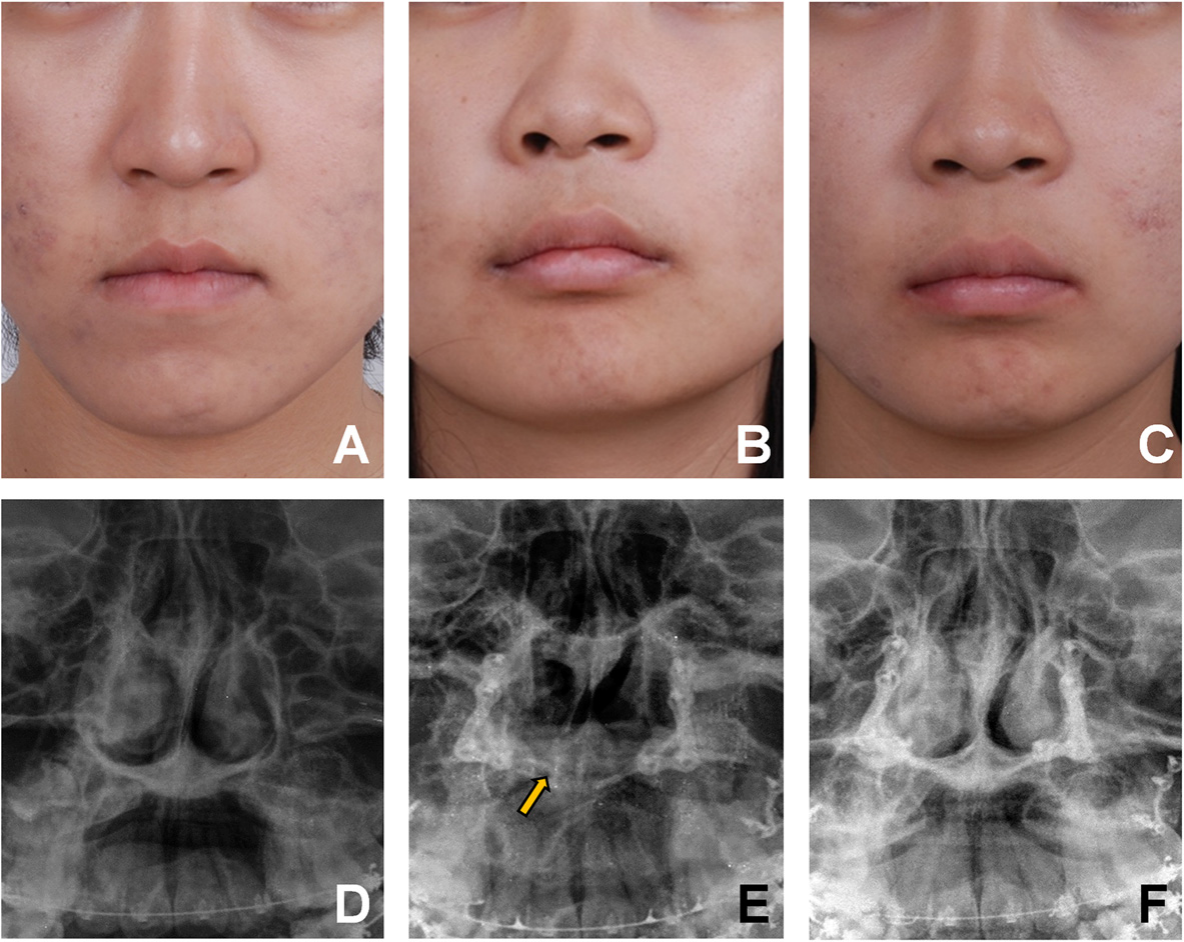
Presurgical condition of case 2 patient before Le Fort I osteotomy (a, d), immediate after the Le Fort I osteotomy (b). Deviated nasal septum (*arrow*) was visible in radiographic examination (e). After correction of nasal septum deviation (c, f). The patient showed corrected septal deviation after the treatment
